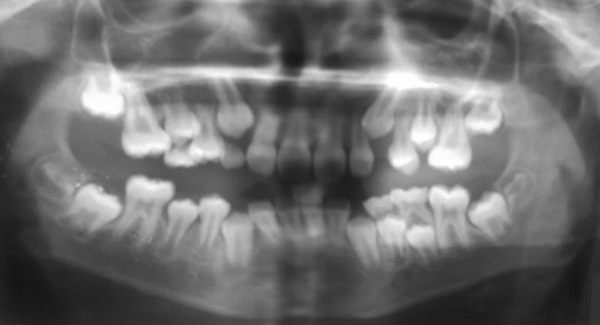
Preoperative orthopantomogram with space deficiency for eruption of both 25 and 15
